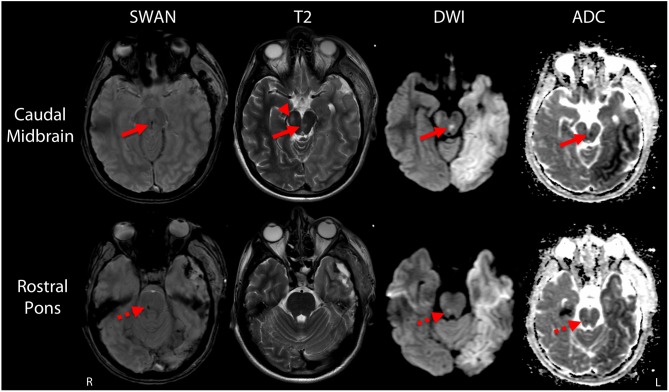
Duret hemorrhages due to traumatic transtentorial herniation. Representative axial images are shown at the levels of the caudal midbrain (top row) and rostral pons (bottom row). In the caudal midbrain, a Duret hemorrhage is indicated by curvilinear hypointense signal at the midline on the susceptibility-weighted angiography (SWAN) image, which corresponds with a hyperintensity on the T2-weighted image (T2) and diffusion-weighted image (DWI), as well as a hypointensity on the apparent diffusion coefficient (ADC) map (solid arrows). A second hypointense lesion is seen in the rostral pons on the SWAN image. The signal properties of this second lesion are similar on the diffusion-weighted image and apparent diffusion coefficient map (dashed arrows), but no corresponding hyperintensity is seen on the T2-weighted image. A T2-hyperintense lesion within the right cerebral peduncle (arrow head) at the level of the caudal midbrain likely represents injury to the descending corticospinal tract fibers due to compression against the tentorium during the herniation event.
